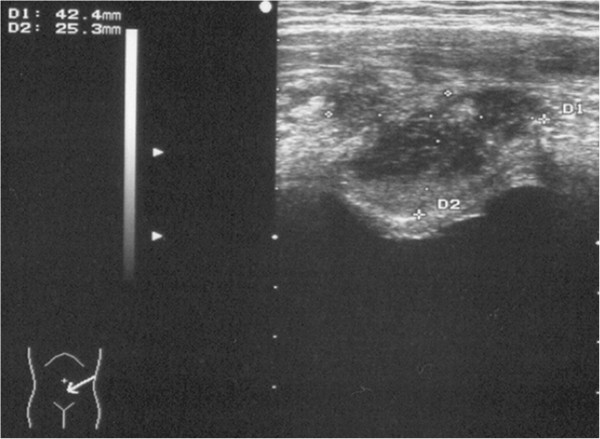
Abdominal ultrasonographic image demonstrating an urachal cystic mass.
